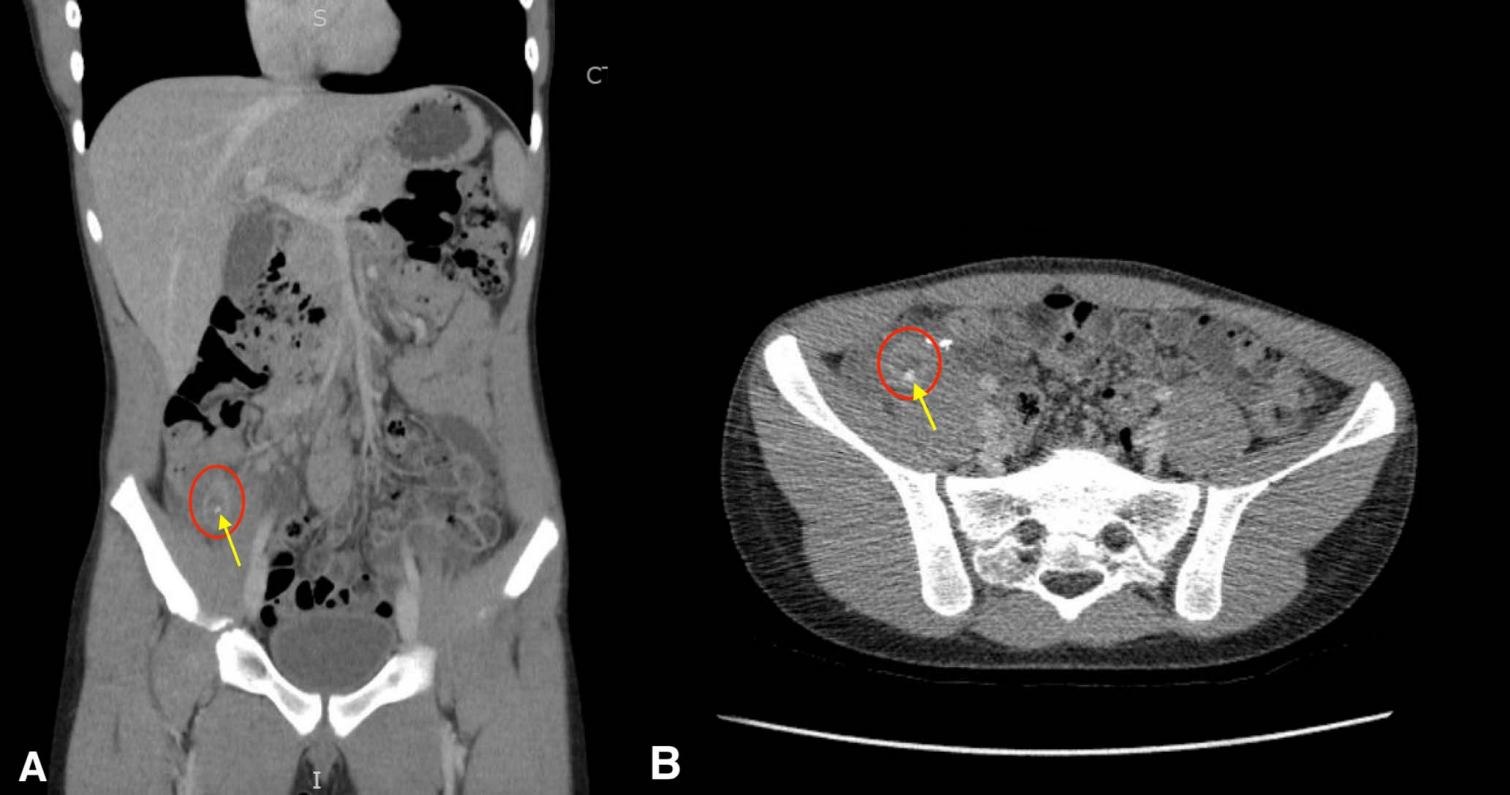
Computed tomography with contrast (A) coronal and (B) axial views of the abdomen and pelvis show the inflamed structure in the region of the previous appendectomy with possible fecalith. The red circles surround the lesion, and the yellow arrows point to fecalith.
